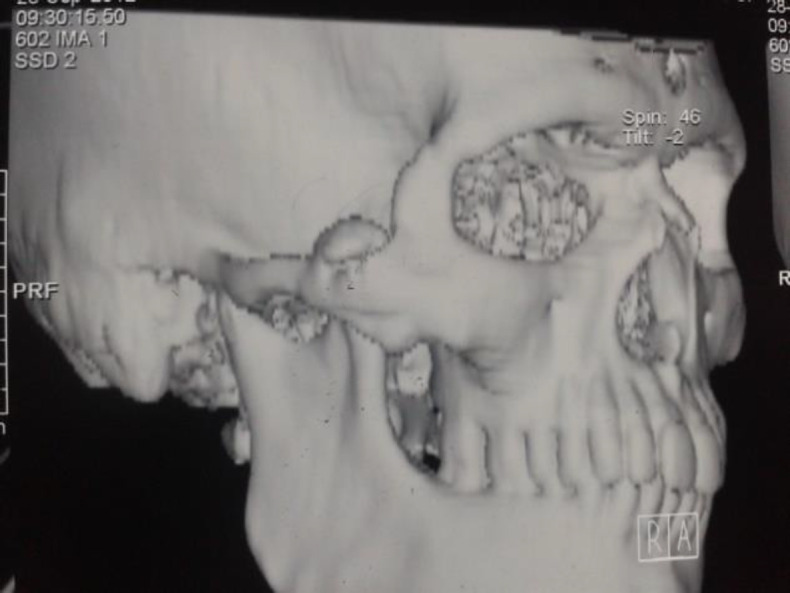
Computed tomography with 3D reconstruction, hyperplastic coronoid posterior to zygomatic arch with pseudojoint formation
